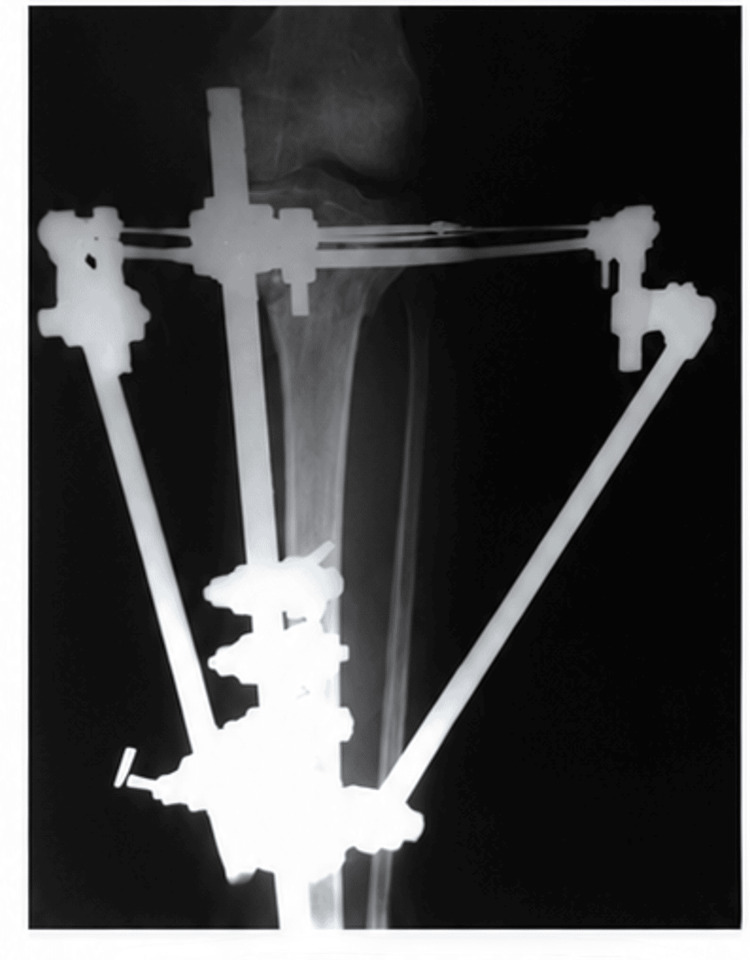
Postoperative radiographic image of a patient with hybrid external fixator fixation
